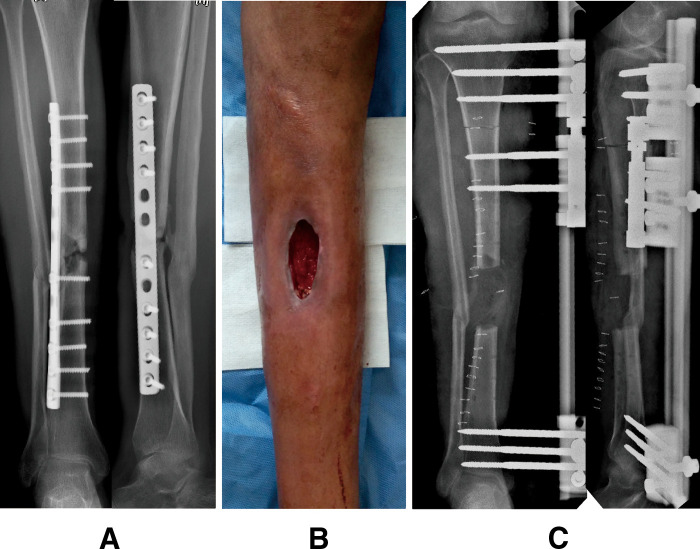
A 42-year-old man who suffered chronic osteomyelitis in his right tibia after internal fixation treatment followed by a road traffic accident and successfully treated by the bifocal bone transport (BFT) technique from proximal to distal. (A) Preoperative anteroposterior (AP) and lateral radiographs. (B) Preoperative general appearance, showing soft tissue defects with drainage and sinus. (C) AP and lateral radiographs immediately after radical debridement and installation of the monolateral external fixator; there were 6-cm bone defects. A BFT technique from proximal to distal was performed to reconstruct the injured limb.
